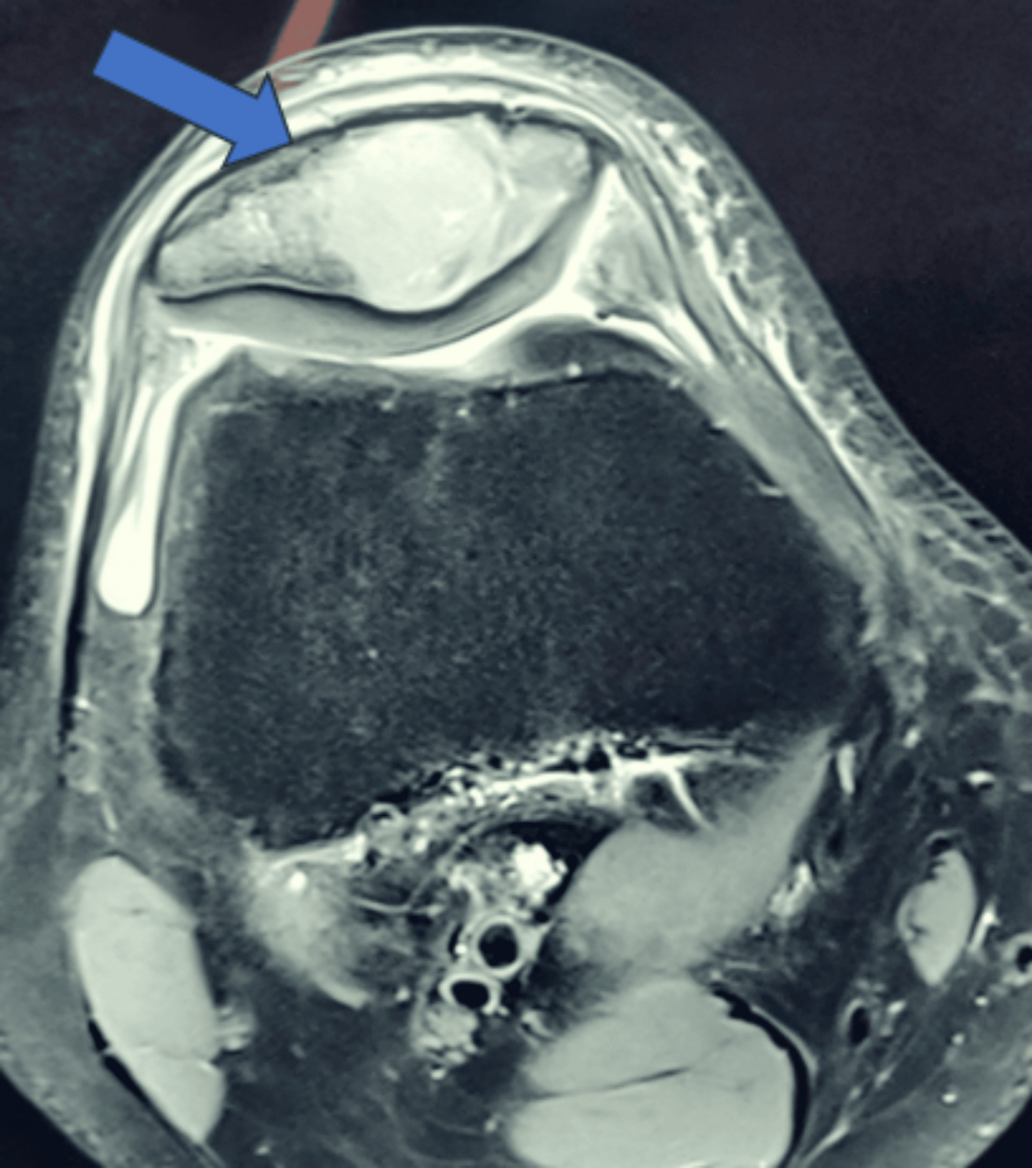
Heterogeneous signal intensity on the T2‑weighted MRI scan, showing heterogeneous signal intensity with an intraosseous lesion in the patella (blue arrow) MRI: magnetic resonance imaging
